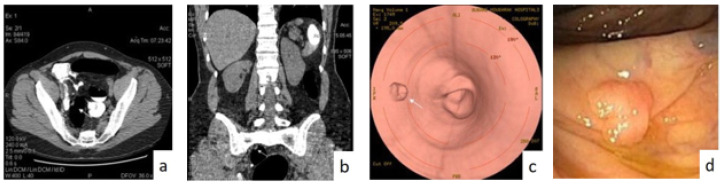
Different images of CT colonoscopy: (a) Axial. (b) Sagittal images. (c) Image of virtual colonoscopy. (d) Image colonoscopy showing the polyp with true-positive findings.
